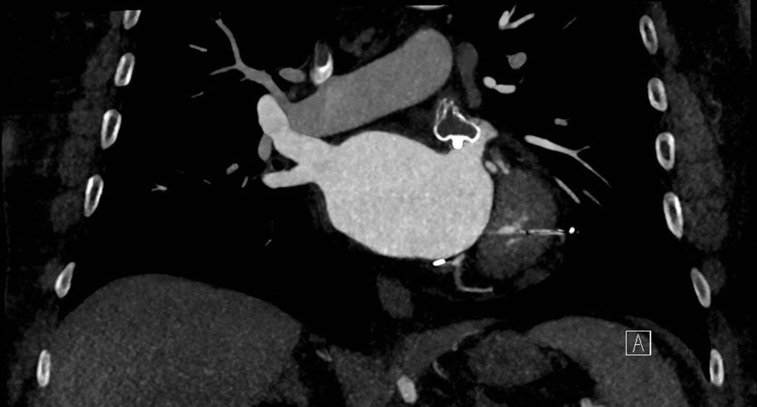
A CT scan demonstrating a large leak around a WATCHMAN^™^ (Boston Scientific, Inc., Natick, MA, USA) device.
